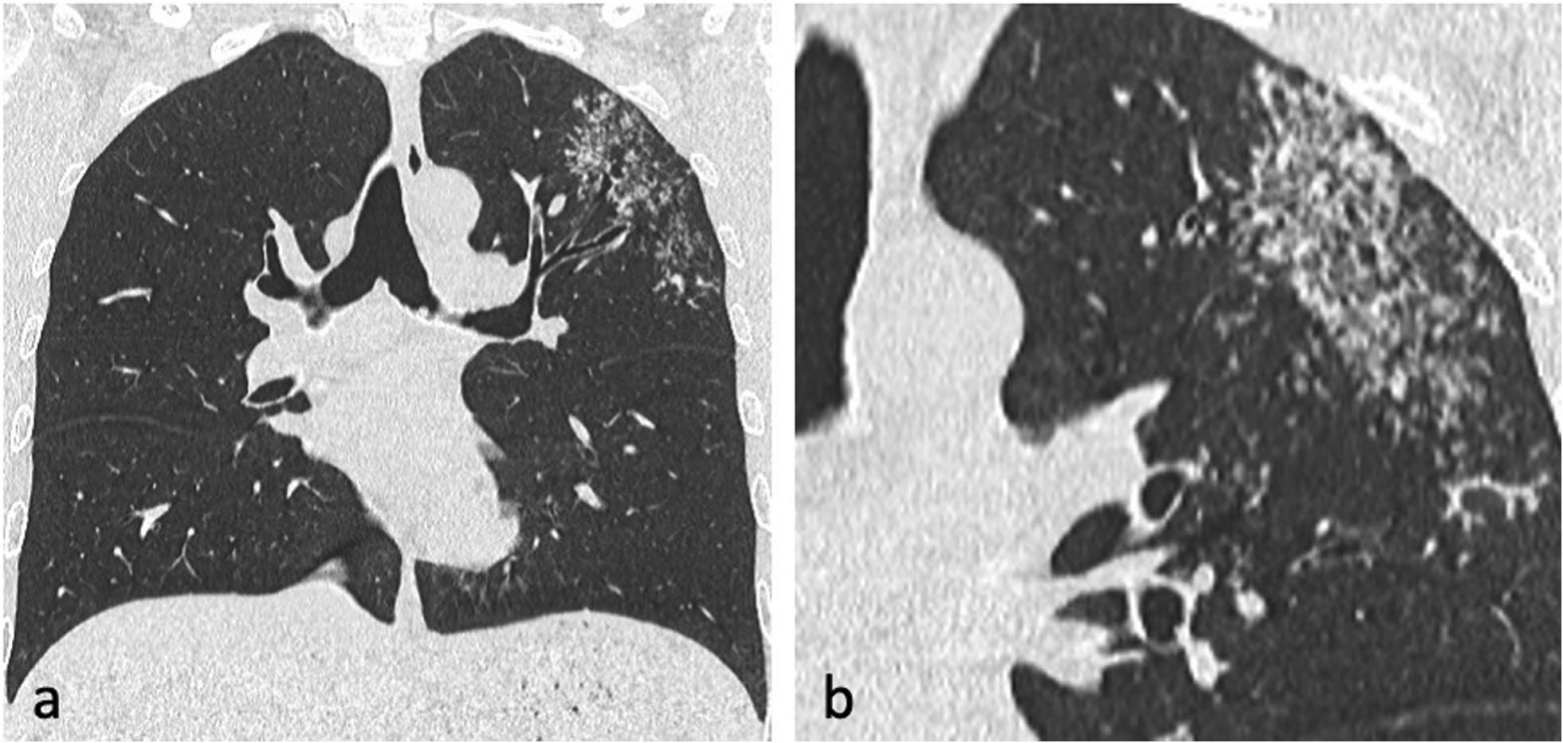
A 29-year-old male presented with cough and haemoptysis. CT (lung window, coronal planes—a, b) demonstrates a cluster of tiny nodules within the apicoposterior segment of the left upper lobe with perilymphatic distribution
